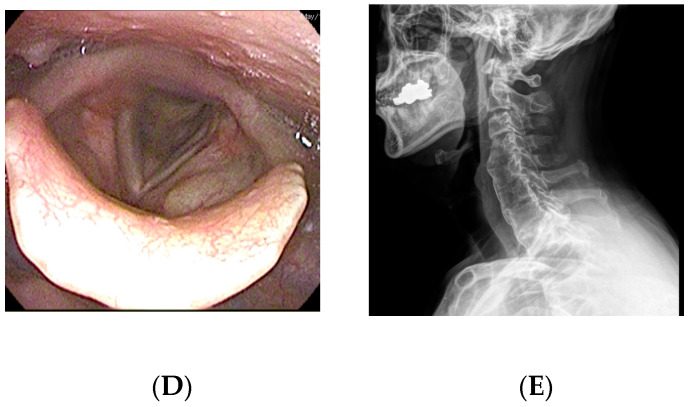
A 64-year-old man presented with swallowing difficulty for the past 2 months. (A) Preoperative laryngoscopy revealed a huge protruding mass at the posterior pharyngeal wall. (B) Lateral cervical radiograph showed continuous irregular hyperostosis along the anterior aspect of the cervical and upper thoracic vertebral bodies, suggesting diffuse idiopathic skeletal hyperostosis (DISH), with a beak-shaped osteophyte compressing the posterior pharynx. (C) Preoperative visual fluoroscopic swallowing study revealed moderate dysphagia in the oral and pharyngeal phases with incomplete closure of the epiglottis. (D) Postoperative laryngoscopy showed disappearance of the protruding mass at the posterior pharyngeal wall. (E) One-year postoperative radiograph showed no evidence of regrowth of the osteophyte.
